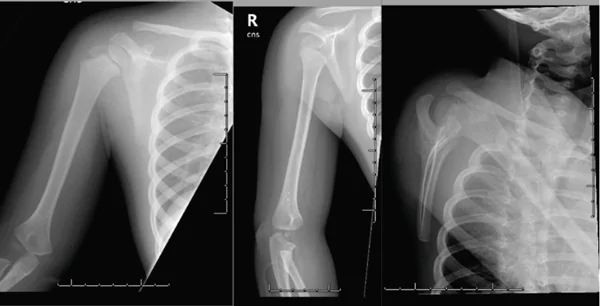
Radiographs of the humerus obtained on admission demonstrating no obvious signs of osseous involvement of the right shoulder.
